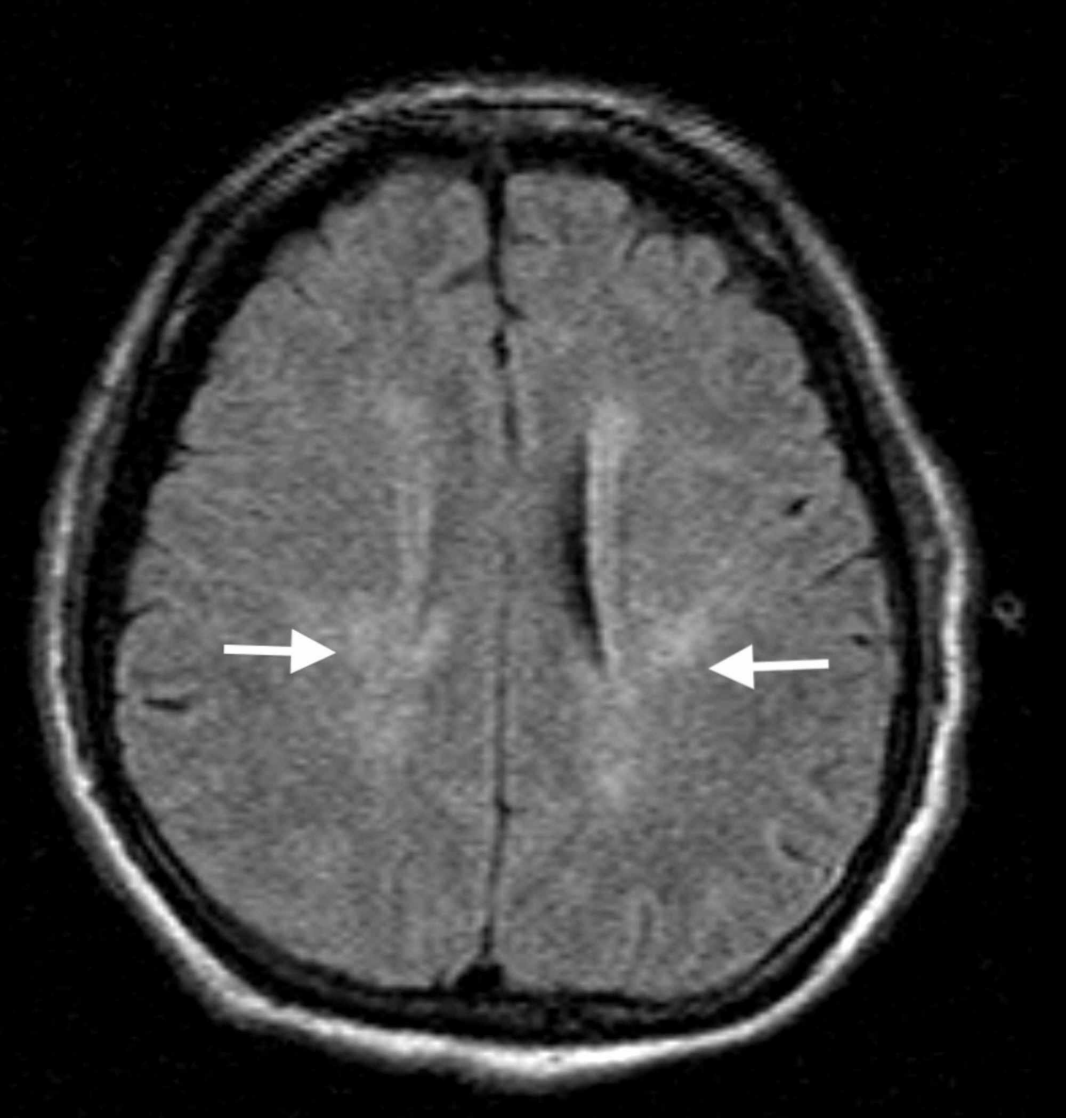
Magnetic resonance imaging of the brain without contrast demonstrating periventricular white matter signal abnormality (white arrows) consistent with chronic ischemic white matter disease versus demyelinating disease.
